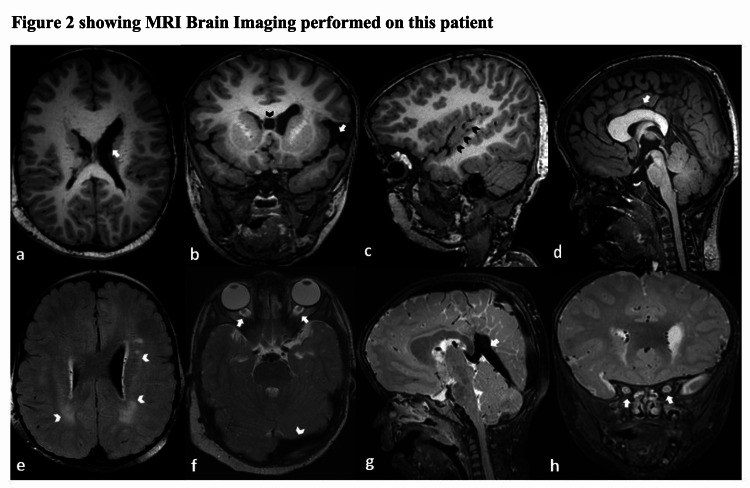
showing MRI Brain Imaging performed on this patient Figure 2 shows MRI Brain imaging performed on this patient. Axial T1W image reveals ventricular asymmetry with prominent left lateral ventricle (white arrow, Fig. 2a). Coronal T1W image reveals left-sided incomplete opercularization with widened left Sylvian fissure (white arrow, Fig. 2b), cavum septum pellucidum with prominent left lateral ventricle is also seen (black arrowhead Fig. 2b).T1W parasagittal image reveals perisylvian polymicrogyria (black arrowheads, Fig. 2c). T1W mid-sagittal image reveals abnormally thickened mega corpus callosum (white arrow, Fig. 2d). Axial FLAIR image demonstrates multiple foci of abnormally increased signals in bilateral deep and periventricular white matter (white arrowheads, Fig. 2e). Axial T2W image reveals prominent bilateral optic nerve sheaths (white arrows, Fig. 2f) and enlarged left transverse sinus flow void (white arrowhead, Fig. 2f). T2W mid-sagittal image reveals an enlarged straight sinus flow void (white arrow, Fig.2g). Coronal T2W image reveals prominent bilateral optic nerve sheaths (white arrows, Fig. 2h).
